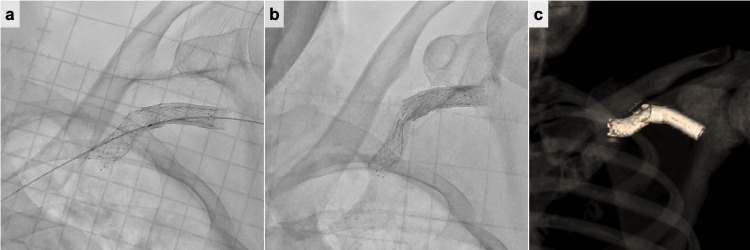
The digital subtraction angiography (DSA) and CT findings. (a) The balloon-expandable covered stent was placed to overlap the damaged stent. (b) Follow-up DSA showed recurrent stent stenosis. (c) 3D-CT revealed the stent fracture. DSA, digital subtraction angiography; CT, computed tomography
